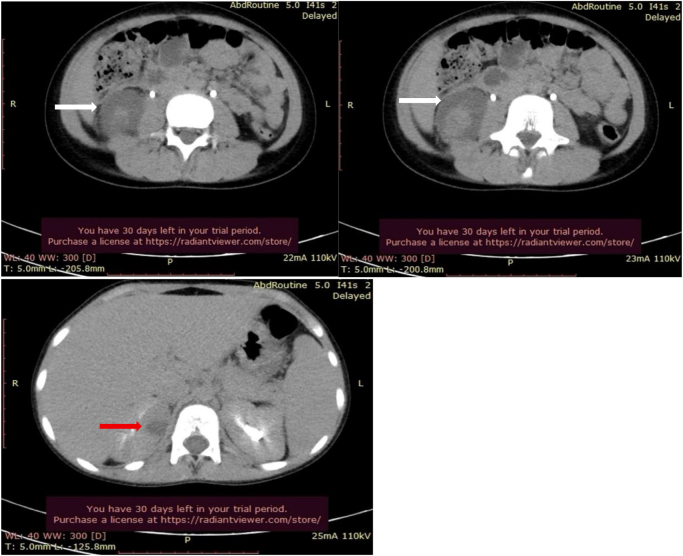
Abdominopelvic CT scan with IV contrast done revealed a 4-mm cortical micro abscess in the upper pole (red arrow) and a 4-cm abscess in the lower pole (white arrow).
